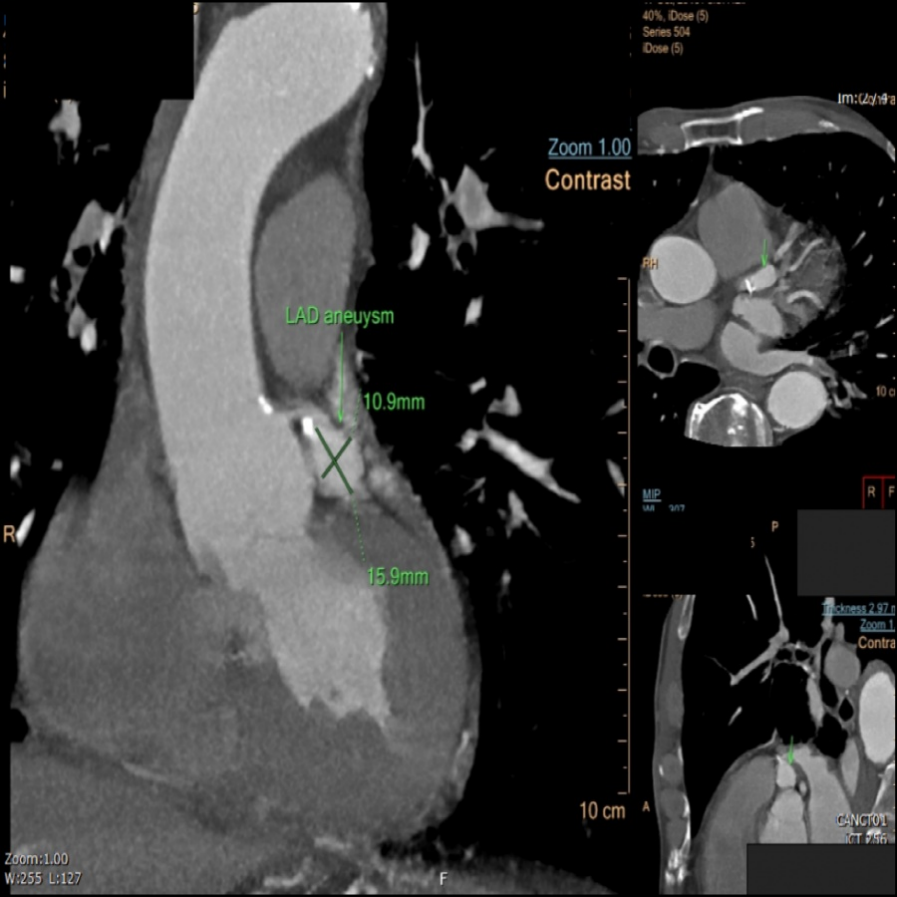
Computed tomography angiogram heart coronary showing aneurysm in the LAD coronary artery (green arrow).
